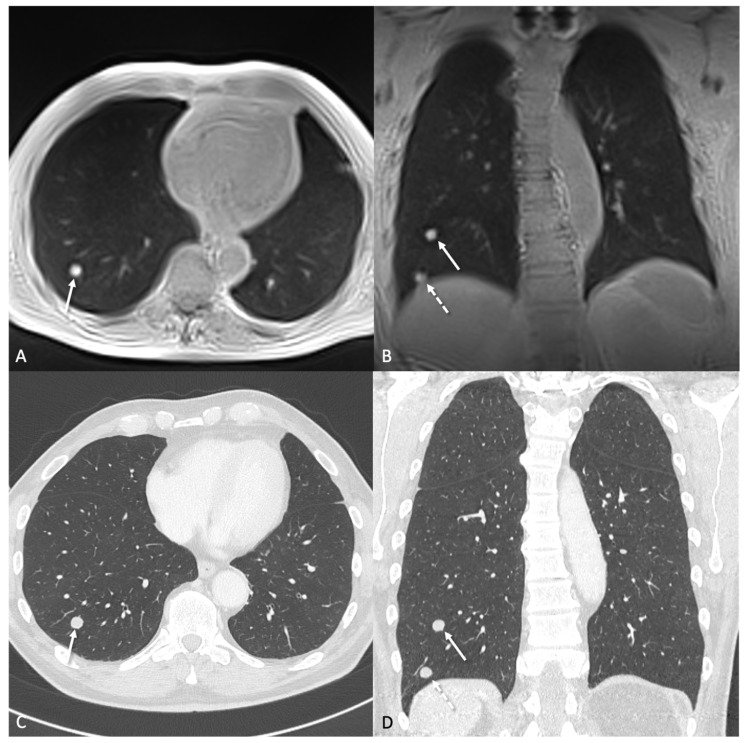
A 76-year-old patient with transitional cell carcinoma. The transversal (A) and coronal (B) ultra-short echo time (UTE) sequence show a 11.3 × 9.8 mm metastasis (arrow) in the right lower lobe. The coronal planes (B,D) additionally show another 11.9 × 9.5 mm pulmonary metastasis next to the right diaphragm (dashed arrow). (C,D) show the corresponding CT at 1 mm slice thickness obtained on the same day.
